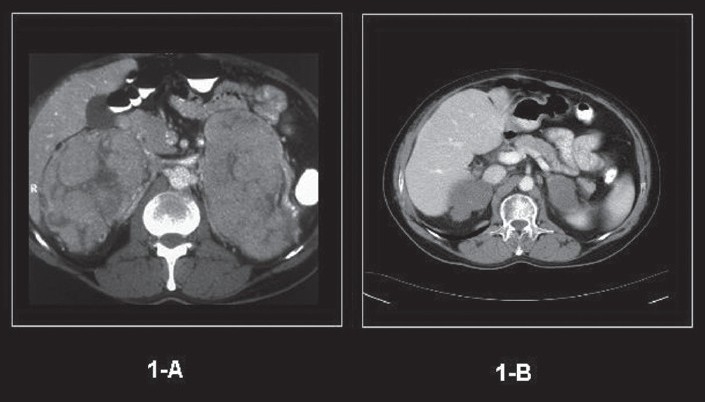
(A) CT scan abdomen, axial view following IV contrast administration (at presentation) revealed bilateral adrenal tumors with heterogeneous enhancement. The right adrenal tumor measured 11×8 cm while the left one measured 13×8 cm. Prominent vessels are demonstrated around the masses. Note the clear lobulations seperated by hypodense linear areas of probable fibrosis and necrosis within the tumor masses. (B) An axial contrast-enhanced CT image through the adrenals performed 47 months follow-up demonstrated marked interval decrease in both size and enhancement of bilateral adrenal masses. The right adrenal tumotr had decreased substantially to 5.2×4×5.7 cm and the left adrenal mass is regressed to 4.5×5×3.5 cm and showed findings of calcification and findings suggestive of fibrosis.
